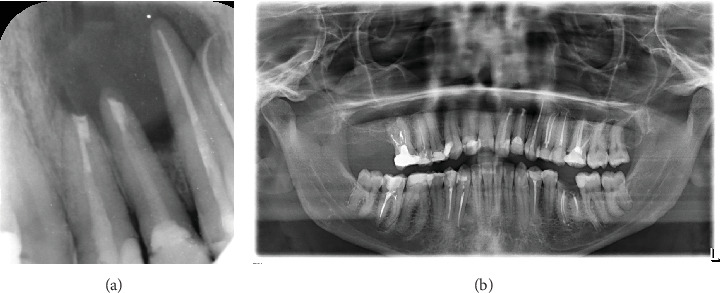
(a, b) 1-year recall radiographic evaluation (periapical and panoramic views).
